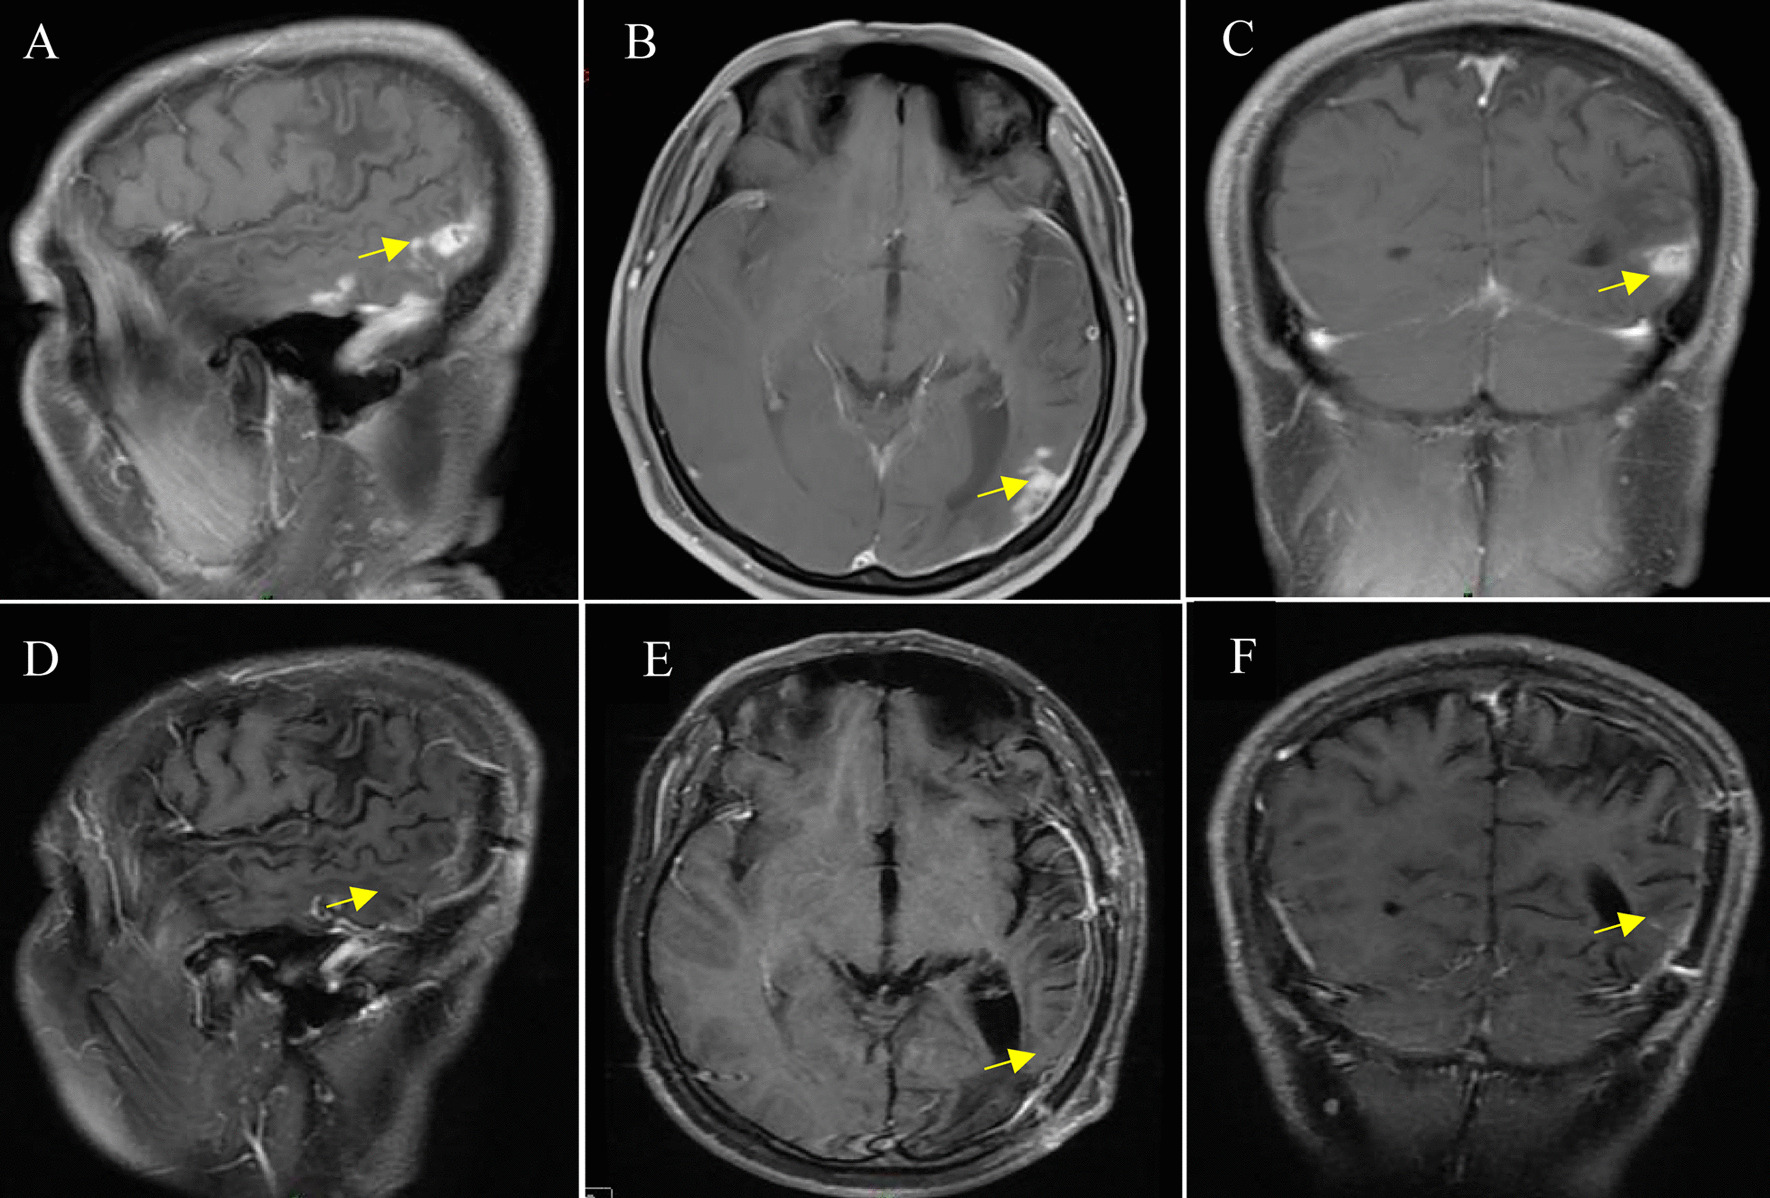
Enhanced MRI of the brain. Preoperative sagittal (A), axial (B), and coronal (C) T1-enhanced MR images show Patchy, nodular and linear enhancements can be seen in the brain parenchyma and adjacent meninges of the left temporoparietal occipital lobe. Postoperative MR images show gross total resection of the Parasite (D–F)
